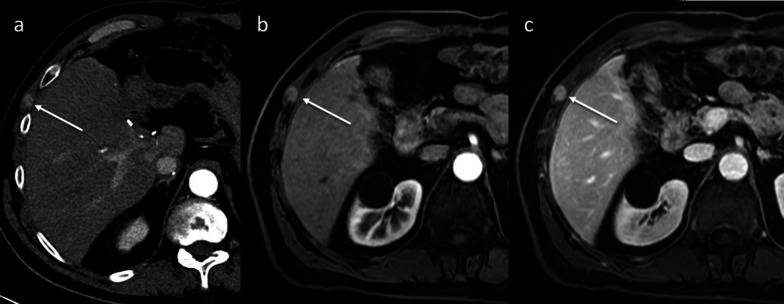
Soft tissue recurrence. Axial contrast-enhanced CT image on the arterial phase (a) exhibits a small muscular recurrence of HCC on the right abdominal wall, showing the typical hypervascular pattern (arrow). This lesion (arrows) is also confirmed on the arterial phase (b) and portal venous phase (c) contrast-enhanced MR images
